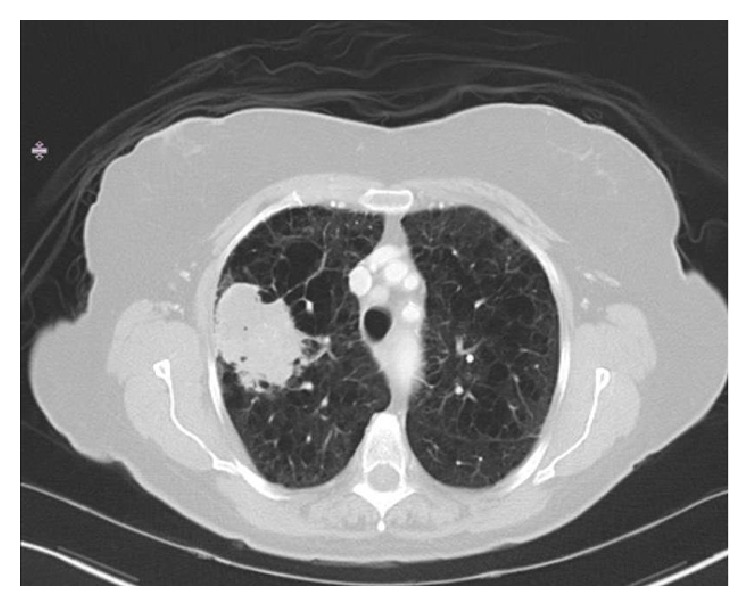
CT chest showing right upper lobe mass with emphysema.
